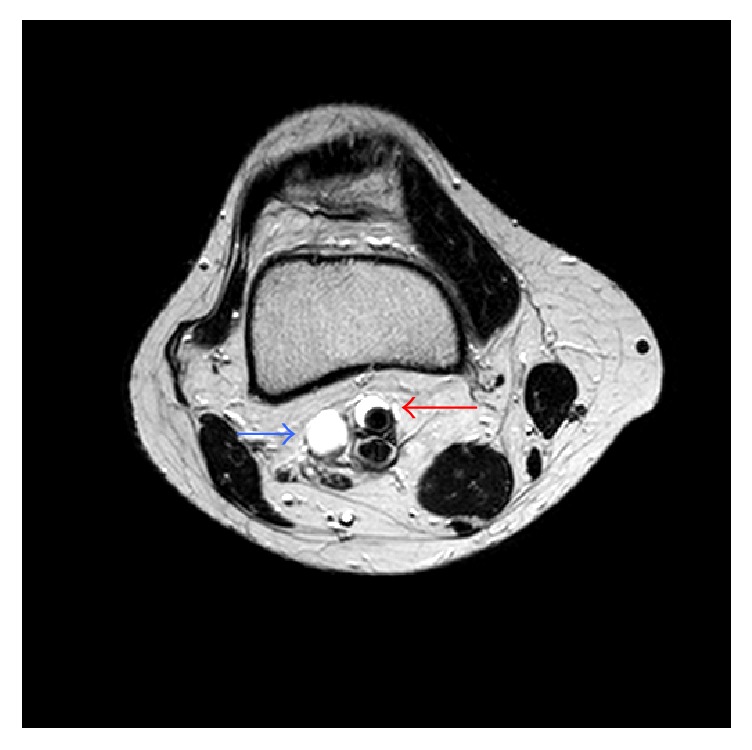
Magnetic resonance imaging (T2-weighted images) revealed cystic lesion encompassing the right popliteal artery circumferentially. Red arrow: cystic adventitial disease of popliteal artery. Blue arrow: venous aneurysm of popliteal vein (it was not diagnosed preoperatively).
